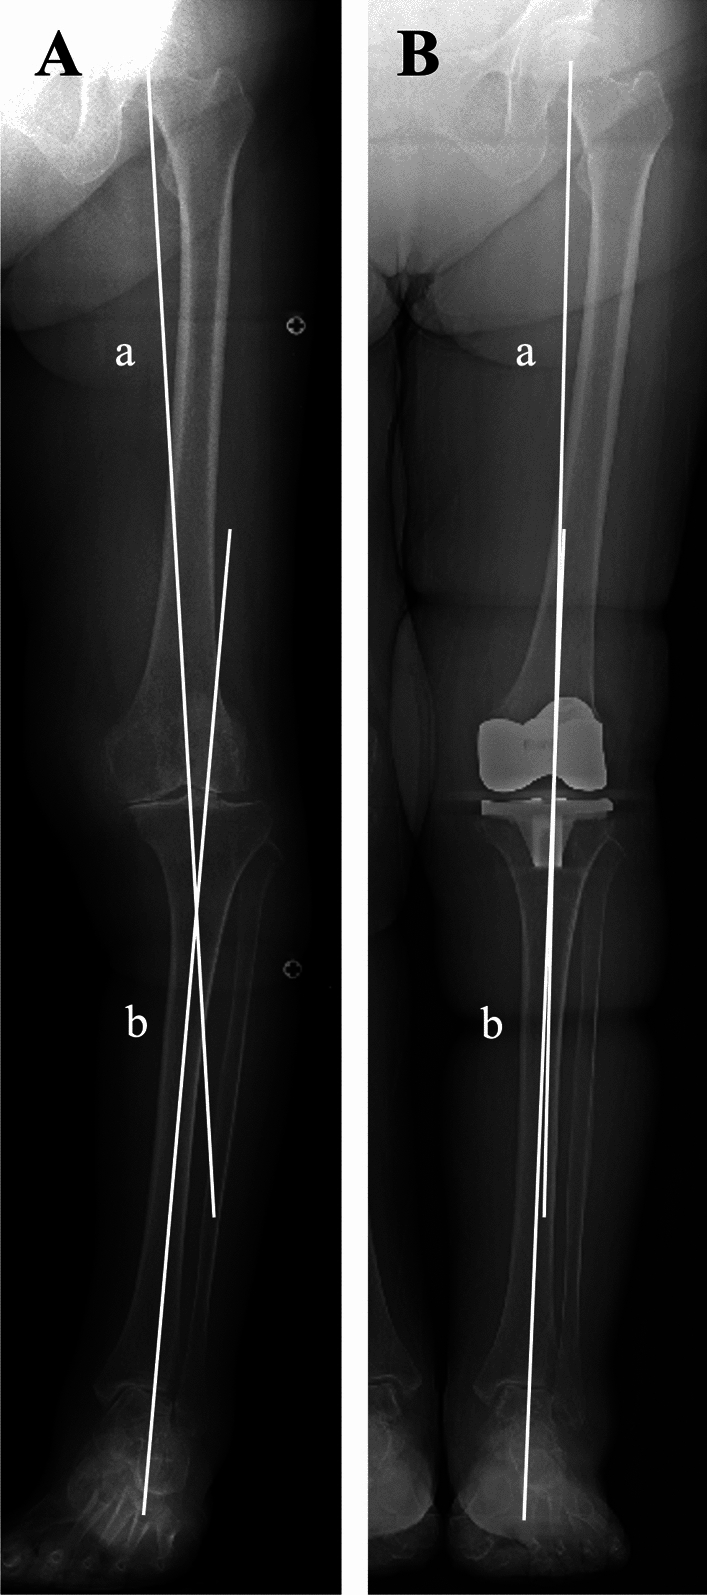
Whole-leg anteroposterior weight-bearing radiographs obtained (A) preoperatively and (B) 2 months after total knee arthroplasty using a mechanical alignment technique. Global alignment of the lower extremity was assessed using the hip–knee–ankle (HKA) angle, defined as the angle between the mechanical axis of the femur (line a) and that of the tibia (line b)
